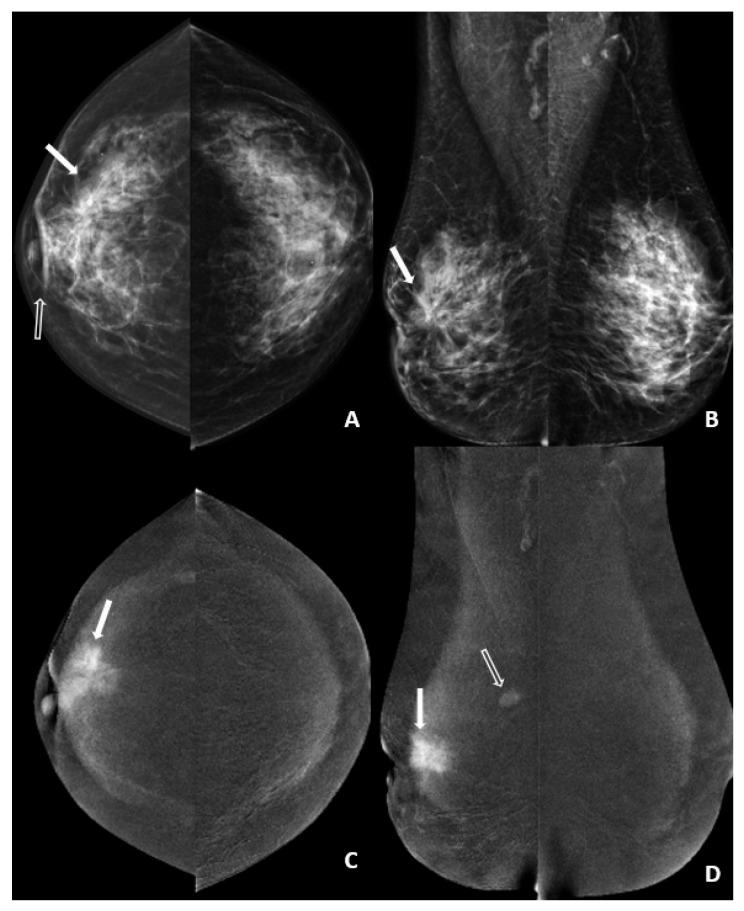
Standard CEM images after CT examination of a 49-year-old female patient with right breast IDC diagnosis. (A,B) Low-energy CC and MLO images show parenchymal distortion area (arrow) in the upper outer quadrant of the right breast with skin and nipple contraction (open arrow). (C,D) Subtracted images show a contrast-enhanced mass with a maximum diameter of 42 mm and an additional enhancing focus in the same quadrant (open arrow). The scores for this patient were as follows: lesion enhancement, 4; conspicuity of the primary lesion, 4; and background parenchymal enhancement, 1.
